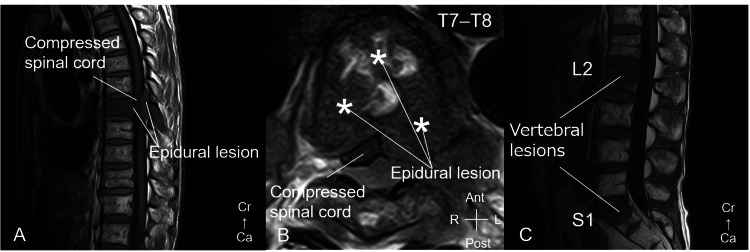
T2-weighted magnetic resonance imaging findings Orientation markers are defined as follows: Cr, cranial; Ca, caudal; Ant, anterior; Post, posterior; R, right; and L, left. A: Sagittal thoracic image showing an epidural lesion at the seventh to eighth thoracic vertebral levels with spinal cord compression. B: Axial thoracic image at the seventh to eighth thoracic vertebral levels showing spinal cord compression by the epidural lesion. Asterisks indicate the epidural lesion. C: Sagittal lumbar image showing additional vertebral lesions at the second lumbar and first sacral vertebral levels.
